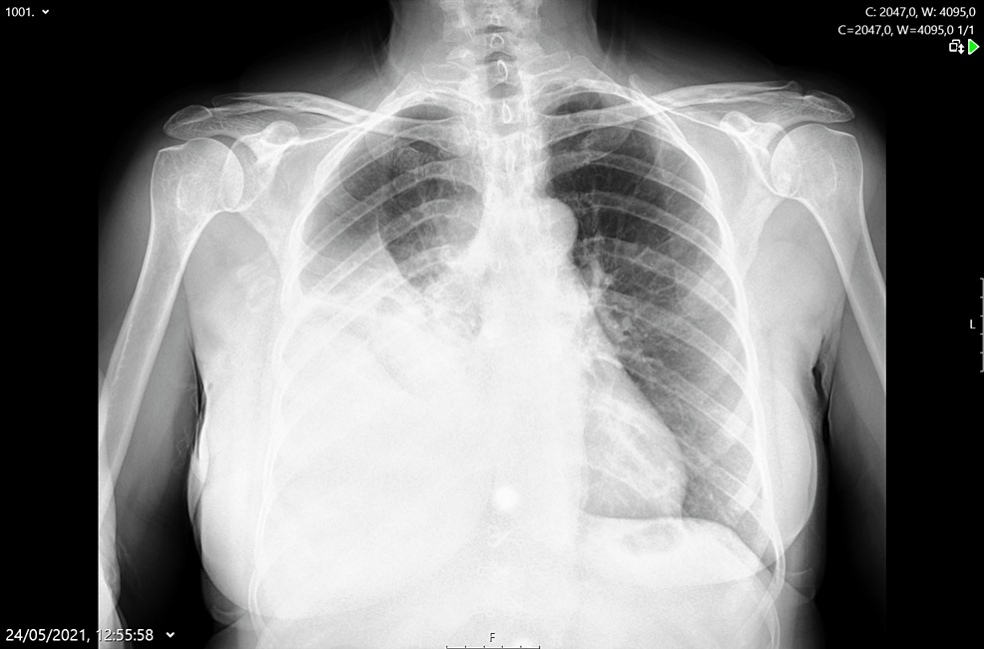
Thoracic X-ray at internal medicine admission Thoracic radiography with medium-sized right-side pleural effusion and disease progression
